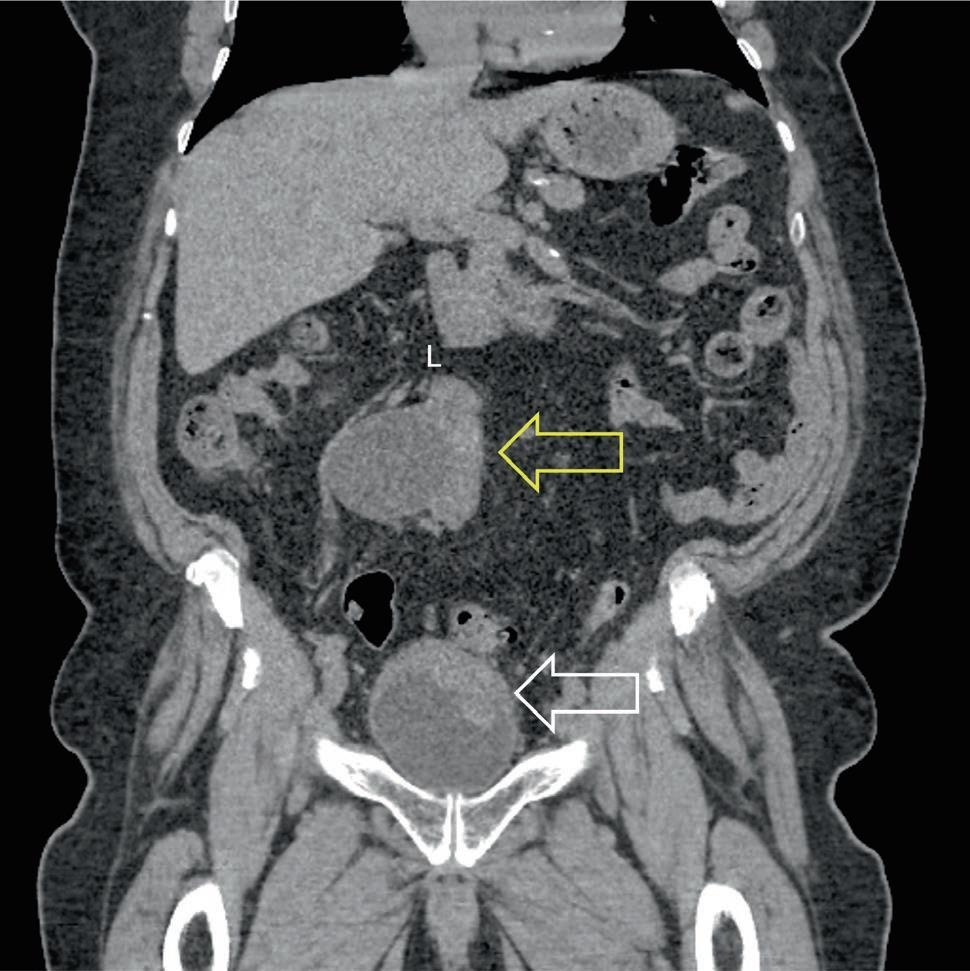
Coronal view of noncontrast CT‐urogram. White arrow indicates muscle‐invasive bladder cancer. Yellow arrow indicates left‐to‐right unfused crossed ectopic kidney.
